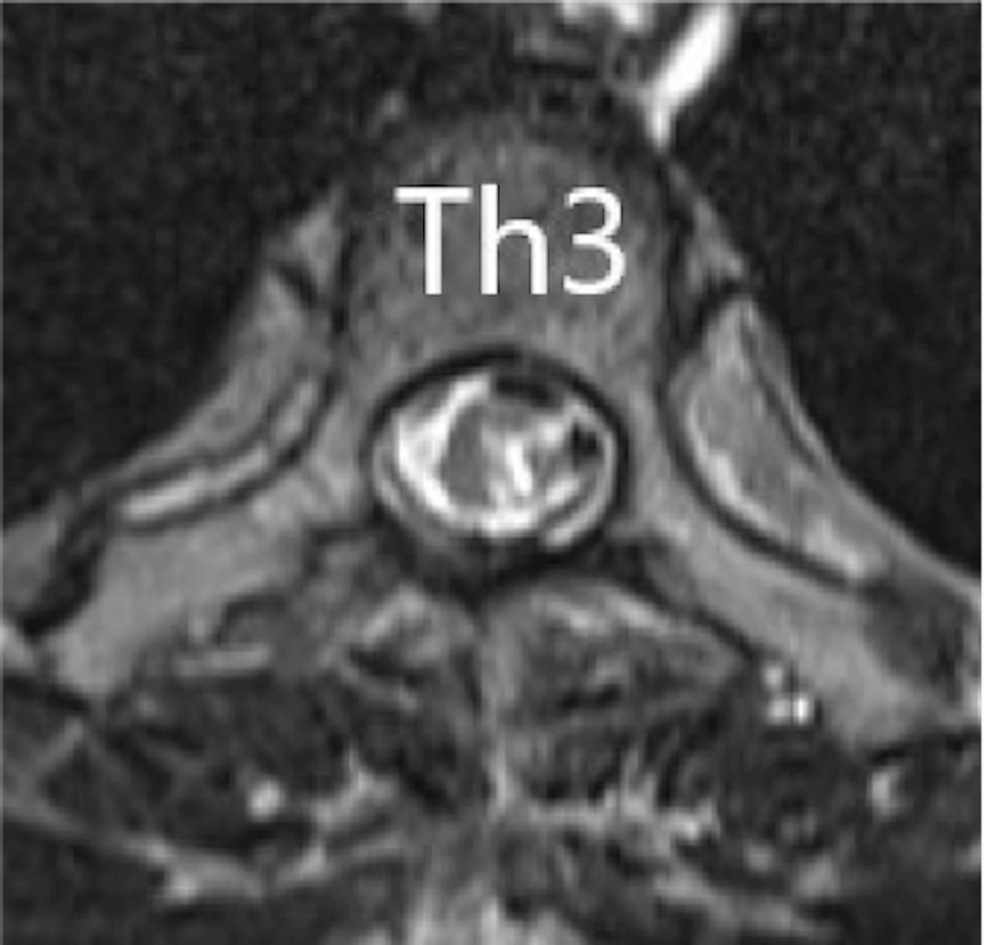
Axial T2-weighted MR images 12 weeks after the operation
